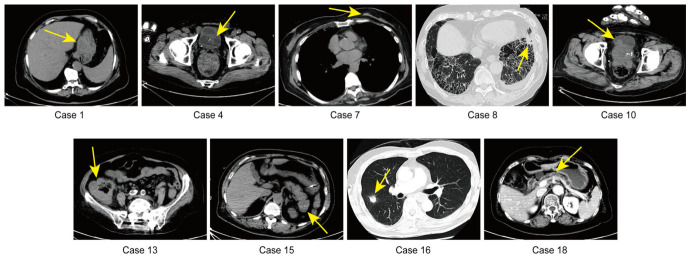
Computed tomography images of the nine incidental malignancies missed in the emergency department. (Case 1) An 81-year-old female presenting with chest discomfort and nausea. CT demonstrates subtle thickening of the upper gastric wall with mild adjacent lymph node enlargement (arrow), subsequently confirmed as gastric carcinoma. (Case 4) A 79-year-old male presenting with back pain and posterior neck pain following a traffic accident. A whole-body CT incidentally demonstrates a protruding lesion with calcification within the urinary bladder (arrow), subsequently confirmed as bladder carcinoma. (Case 7) A 69-year-old female presenting with hemoptysis. CT demonstrates a nodule in the left mammary gland (arrow), subsequently confirmed as breast carcinoma. (Case 8) A 75-year-old male presenting with cough and dyspnea. CT demonstrates an enlarging peripheral nodule in the left pulmonary lingula (arrow), subsequently confirmed as lung carcinoma. (Case 10) An 82-year-old male presenting with fever and fatigue. CT demonstrates a 17 mm elevated lesion at the right bladder neck (arrow), subsequently confirmed as bladder carcinoma. (Case 13) An 82-year-old female presenting with hematochezia. CT demonstrates irregular wall thickening of the cecum (arrow), subsequently confirmed as malignant lymphoma of the ileocecal region. (Case 15) A 69-year-old male presenting with dyspnea. CT demonstrates a solid mass in the pancreatic tail (arrow), subsequently confirmed as pancreatic carcinoma. (Case 16) A 68-year-old male presenting with hematemesis and melena. CT demonstrates a solid nodule in the right upper lobe (arrow), subsequently confirmed as lung carcinoma. (Case 18) A 69-year-old female presenting with right upper quadrant pain. CT demonstrates main pancreatic duct dilation with pancreatic atrophy and multiple cysts (arrow), subsequently confirmed as cholangiocarcinoma.
